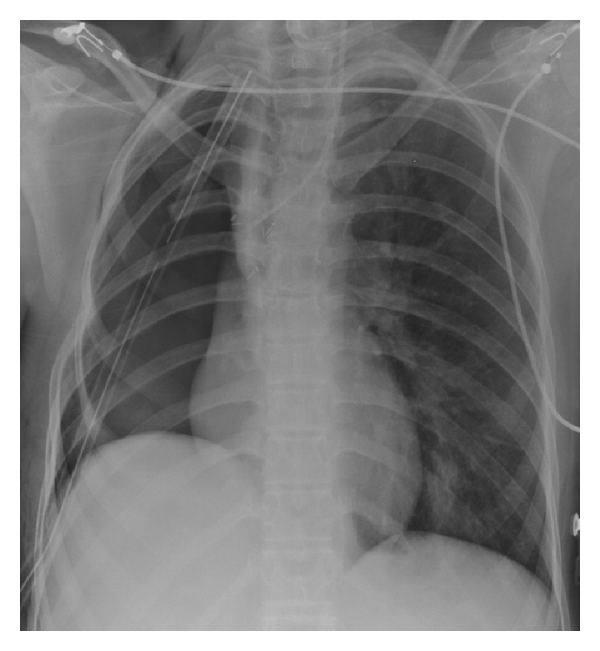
Supine portable chest radiograph obtained 12 hours after resection of the Wilm's metastasis shows the expected early postoperative appearance of the right pneumonectomy space, thoracotomy and bony resection of the 5th right posterior rib, midline position of the mediastinum, and predominantly air in the pneumonectomy space.
